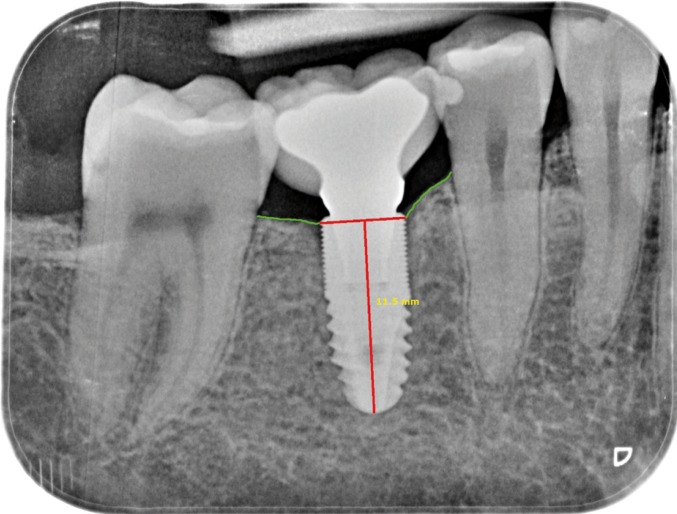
Measurement of peri-implant bone loss with periapical X-ray view after 12 months of follow-up.
